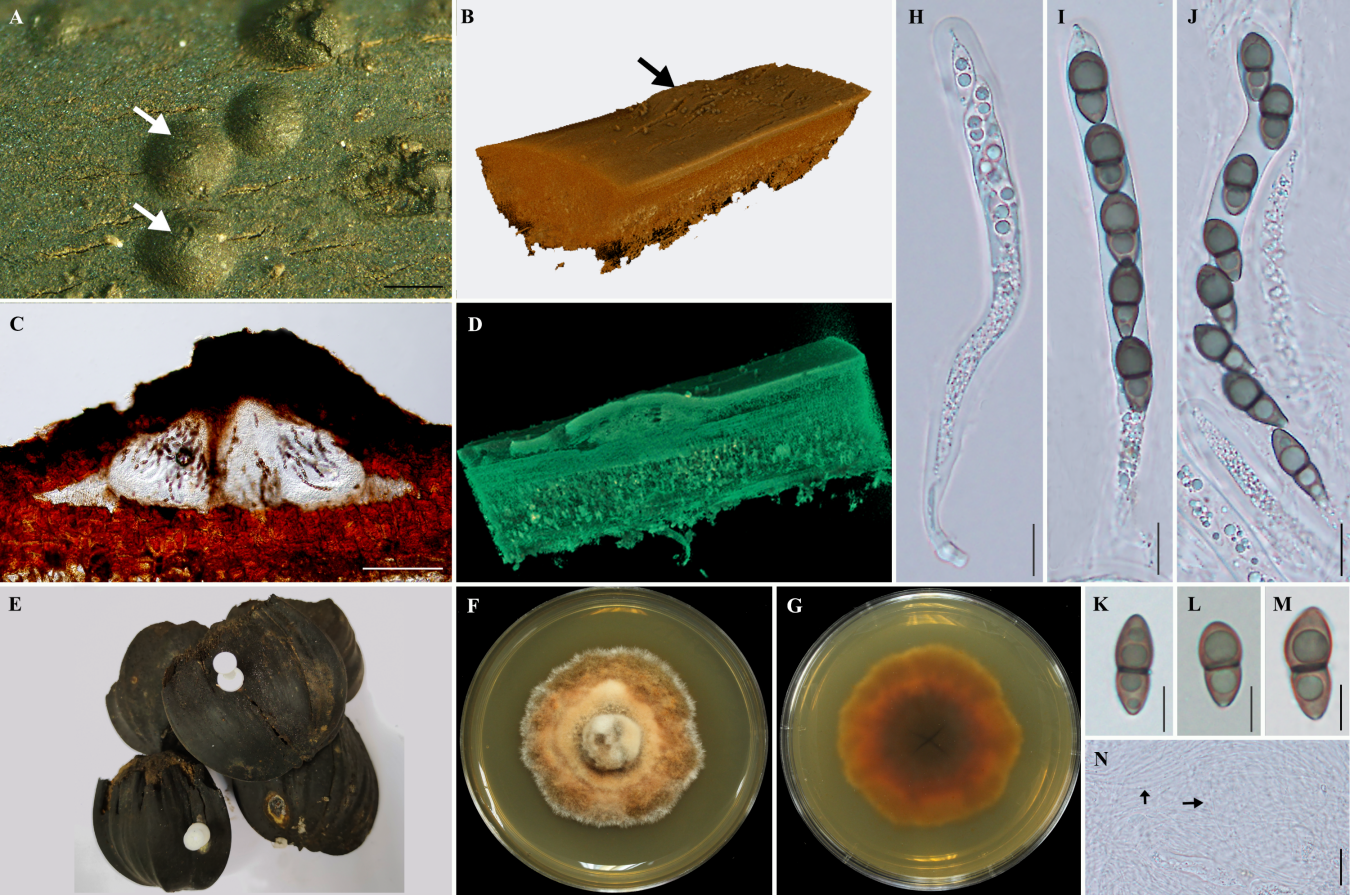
Morphological features of *Helicascus satunensis* sp. nov. (A) Carbonaceous ascomata on the exocarp of *Nypa* palm fruit. (B) The 3D visualization of the ascoma by X-ray tomography (arrow). (C) Vertical section through an ascoma. (D) Section through an ascoma using 3D visualization. (E) Dead *Nypa* palm fruits. (F–G) Obverse and reverse views of cultures grown on SPDA after 60 days. (H–J) Subcylindrical bitunicate asci. (K–M) Ascospores. (N) Pseudoparaphyses (arrow). Scale bars *A* = 1 mm, C =300 µm, H–J = 100 µm, K–M = 10 µm, *N* = 20 µm.
